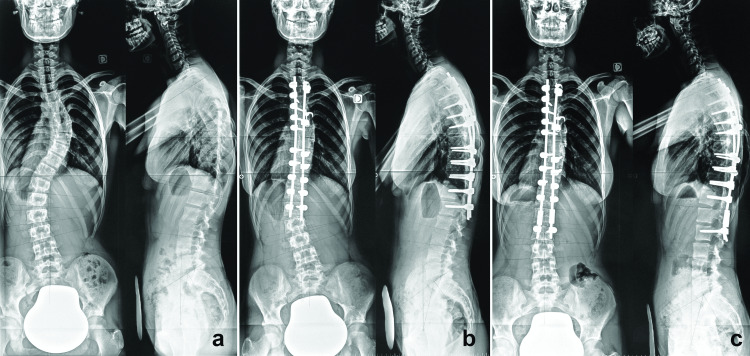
A representative case of the series a) Preoperative postero-anterior and lateral radiographs (Lenke 1C curve). b) Radiographs six months after index surgery (OP1) with a clear global and local imbalance from excessive disc wedging below the LIV. A distal junctional kyphosis is noted. c) Radiographs at 26 months from revision surgery showing constancy of the amelioration.
